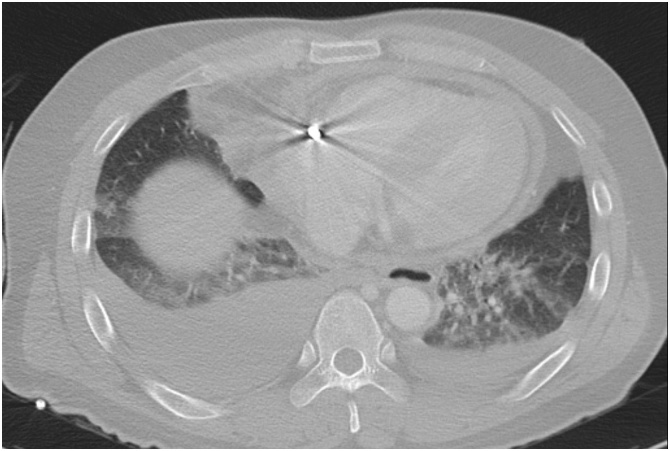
Chest computed tomography scan CT showing hemopericardium and bilateral haemothorax.
